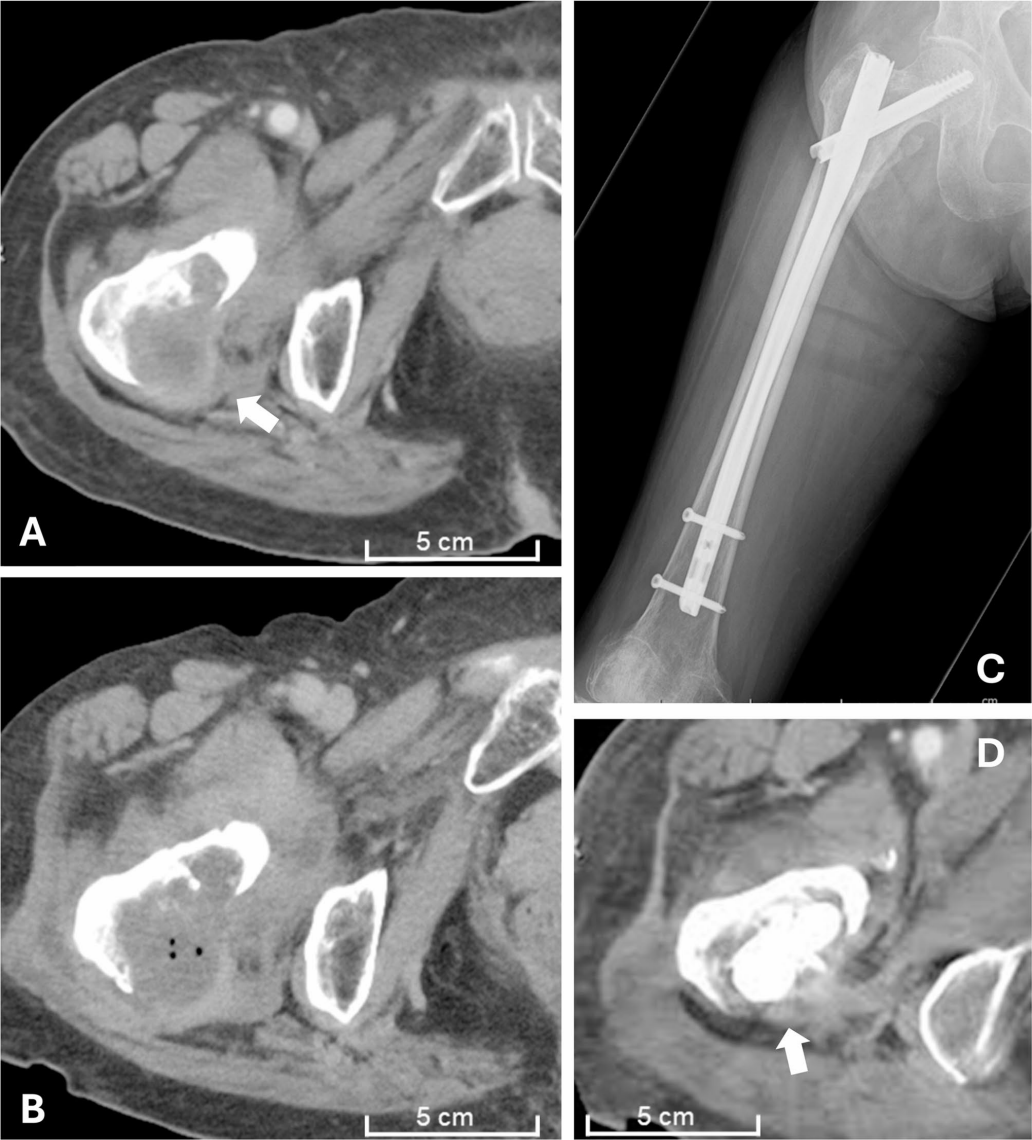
Local control effect of RFA (Case 2). Preablation CT image with contrast enhancement (arrow) (A). CT on the day after RFA showing reduced enhancement and central gas formation (B). Postoperative radiograph (C). Contrast-enhanced CT at 5 months postsurgery showing posterior extraosseous mass reduction without curettage in this area (arrow) (D). RFA: Radiofrequency ablation
